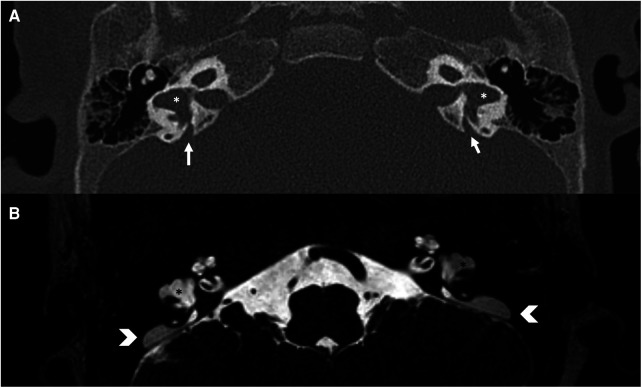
Bone-CT (A) and T2 weighted-MRI (B) imaging of a case of bilateral enlarged vestibular aqueduct (white arrows) and bilateral dysplastic lateral semicircular canals (asterisks). In CT the vestibular aqueduct is enlarged (white arrows), in MRI it is possible to observe the large endolymphatic sac within (white arrowheads) and in the middle cranial fossa.
